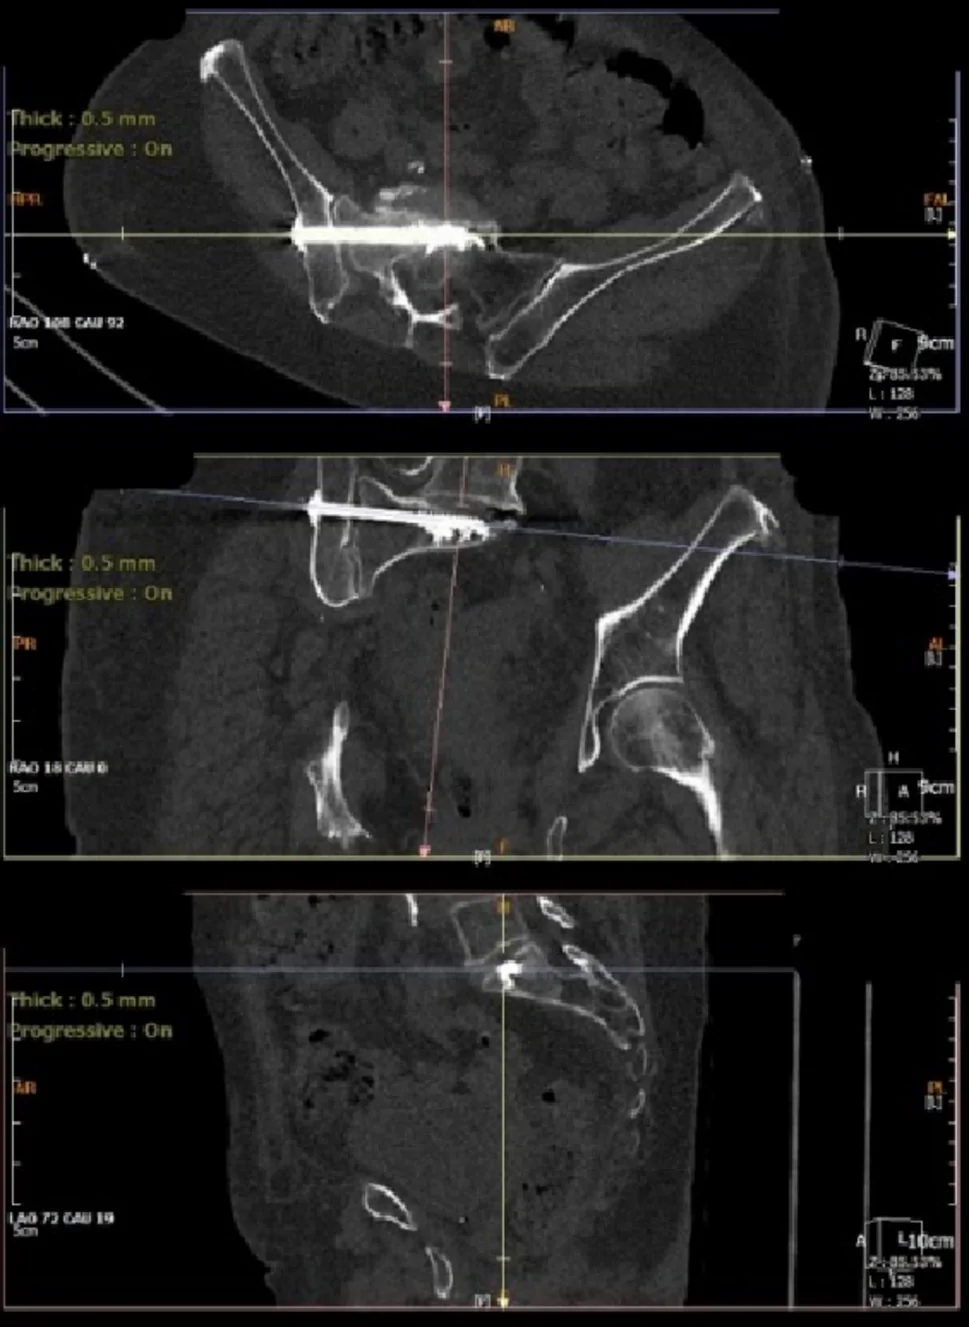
Multiplanar CT reconstruction SI-S1-screw rt.* (Same patient as in figures 10 and 12). Source: Haida et al. (2025), *Die Unfallchirurgie* [16]. CC BY 4.0.
